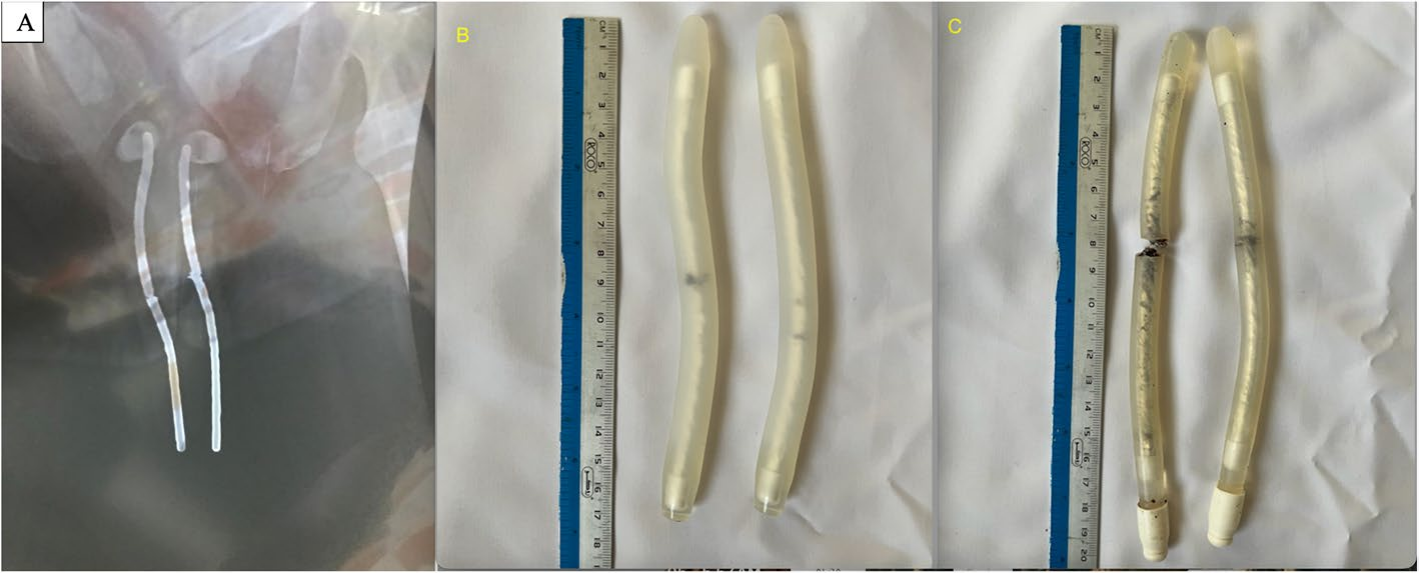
Radiographic and intraoperative findings of fractured malleable penile implants. A Penile anteroposterior X-ray of Patient 1 showing bilateral silver wire fractures. B Explanted penile device from Patient 3, demonstrating separation of silver wires with intact silicone covering. C Explanted penile device from Patient 1, showing separation of both silver wires and the silicone tube on one side
